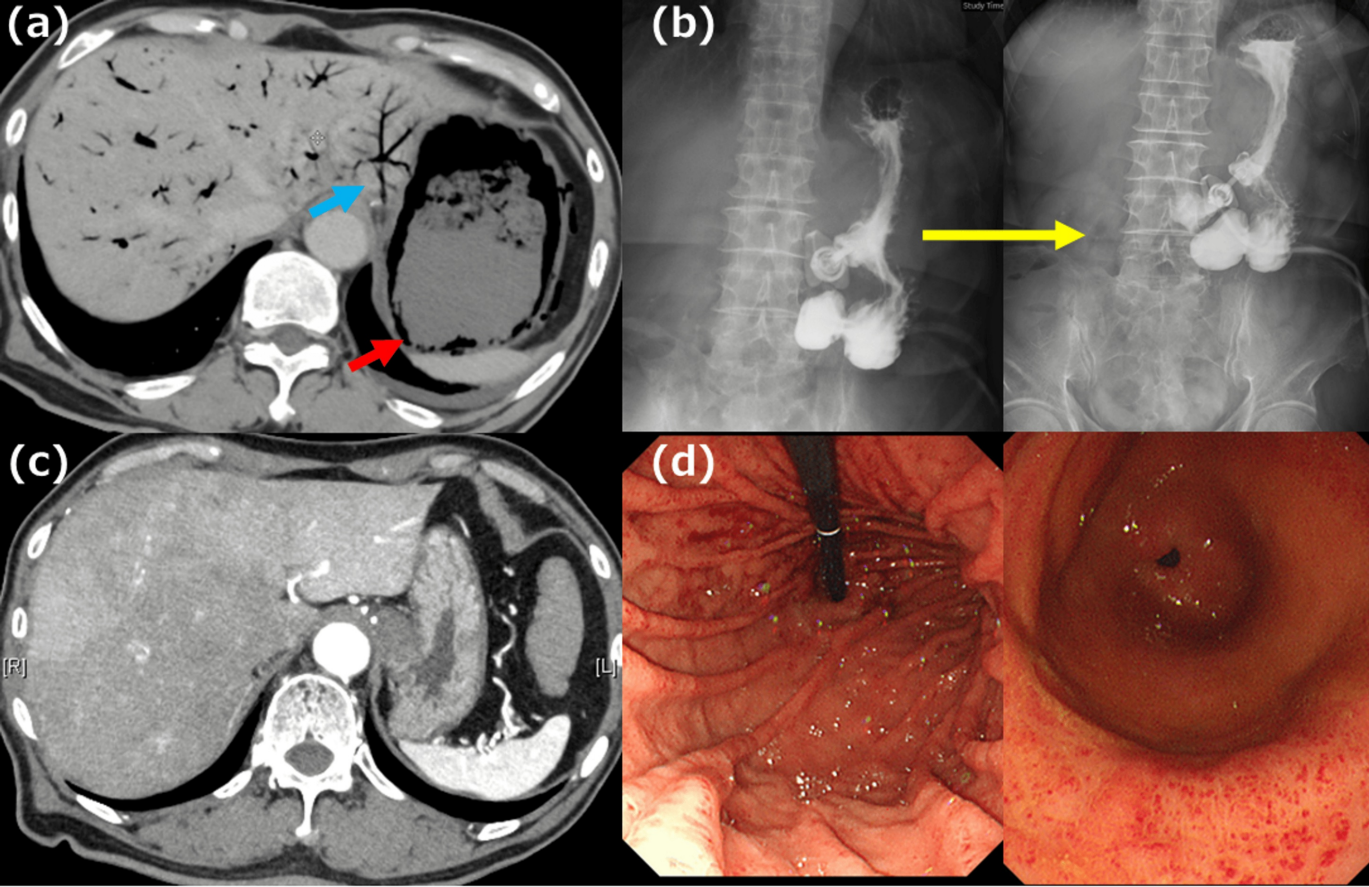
CT, upper GI series, and EGD findings in Case 1 (a) CT showed gastric emphysema (red arrow) and hepatic portal vein gas (blue arrow). (b) Four hours later, Gastrografin🄬 iodinated contrast did not pass the pylorus, indicating DGE. (c) After the sixth day of conservative treatment, gastric emphysema, and hepatic portal vein gas disappeared. (d) There is no erosion of the gastric mucosa and punctate redness is observed in the gastric antrum. No ischemic findings were observed. GI: gastrointestinal; CT: computed tomography; EGD: esophagogastroduodenoscopy; DGE: delayed gastric emptying
